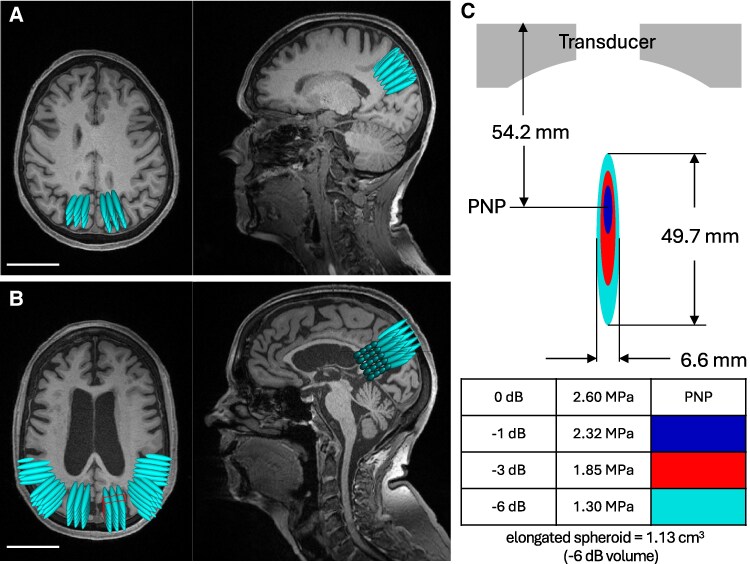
Treatment planning. Volumetric MRI scans from baseline visits were used to plan each participant’s treatment in the Brainsight navigation system. Blue spheroids denote individual targets and represent the −6 dB volume of the ultrasound transducer’s focal region. Axial (left) and sagittal (right) views shown as examples of (A) target volumes for Cohort 1 receiving 30 sonications per treatment visit (30 cm^3^, targeting the bilateral precuneus region) (B) and Cohort 2 receiving 100 sonications per treatment visit (100 cm^3^, bilateral temporo-parietal association cortex). (C) Focus profile measured in free field. MPa, megapascal; PNP, peak negative pressure. Scale bar (A,B): 50 mm.
